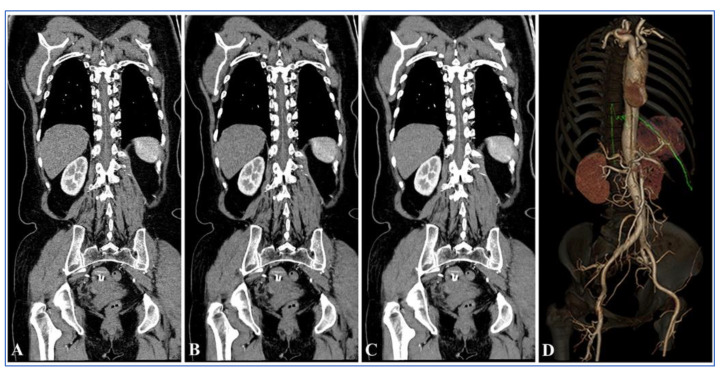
Thoracoabdominal aorta CTA at 100 kV in a 60-year-old female patient. (A–C) Oblique multiplanar reconstruction images obtained along the course of the ASA and AKA. (A) For FBP reconstruction, the subjective image quality score was 3, and the visualization score of the AKA was 3. (B) For ASIR-V 40% reconstruction, the subjective image quality score was 4, and the visualization score of the AKA was 4. (C) For 80% ASIR-V reconstruction, the subjective image quality score was 5, and the visualization score of the AKA was 5. (D) The 3D VR image of the right anterior oblique shows the continuity between the AKA and the aorta, ASA, and the AKA originated at the level of the 10th intercostal artery. A Stanford Type A aortic dissection was revealed, and an intimal tear was located at the plane of the aortic arch, which extended downward to the right common iliac artery. CTA = computed tomographic angiography; ASIR-V = adaptive statistical iterative reconstruction-V; ASA = anterior spinal artery; FBP = filtered back projection; AKA = Adamkiewicz artery; and VR = volume rendering.
